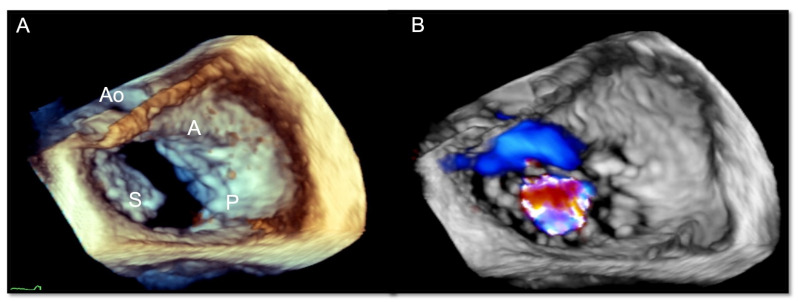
Transesophageal echocardiogram. (A) 3D en face imaging of the tricuspid valve acquired from a mid-esophageal commissural view with Z rotation: this is called the “surgeon’s view” and images the aorta and anterior leaflet (A) on top; (B) with Color Doppler documenting severe tricuspid regurgitation (TR). A = anterior leaflet, S = septal leaflet, P = posterior leaflet.
